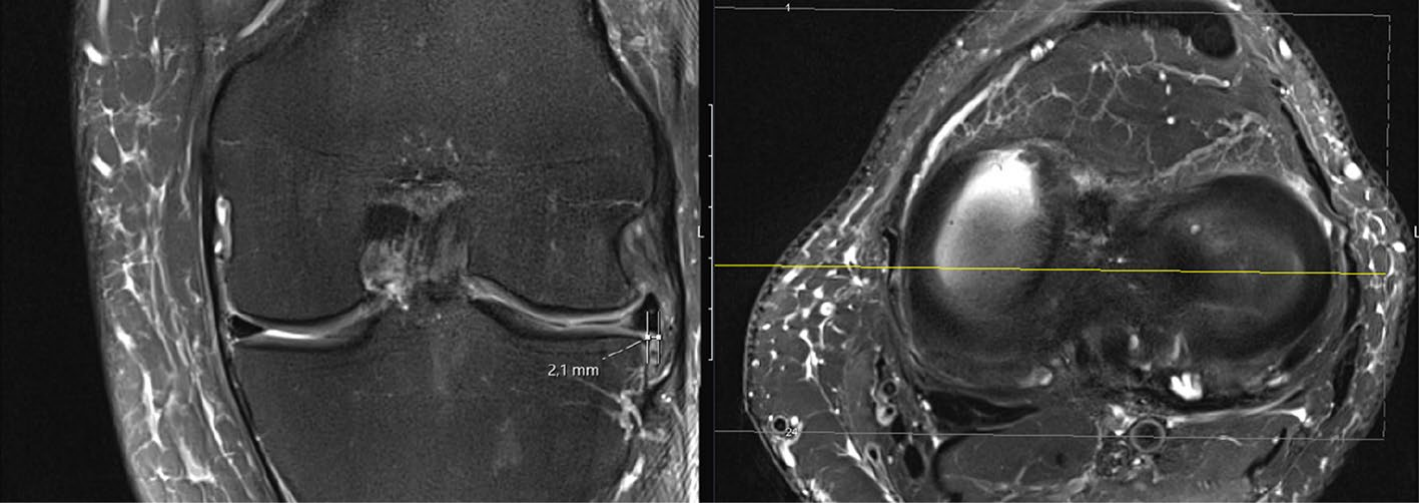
Magnetic resonance imaging. Measuring of the coronal extrusion. The yellow line on the axial plane indicates the position of measuring. According to Ahn et al. [2], the greatest distance of the meniscus from the peripheral margin of the lateral proximal tibial plateau to the meniscocapsular junction was measured on the coronal plane images
